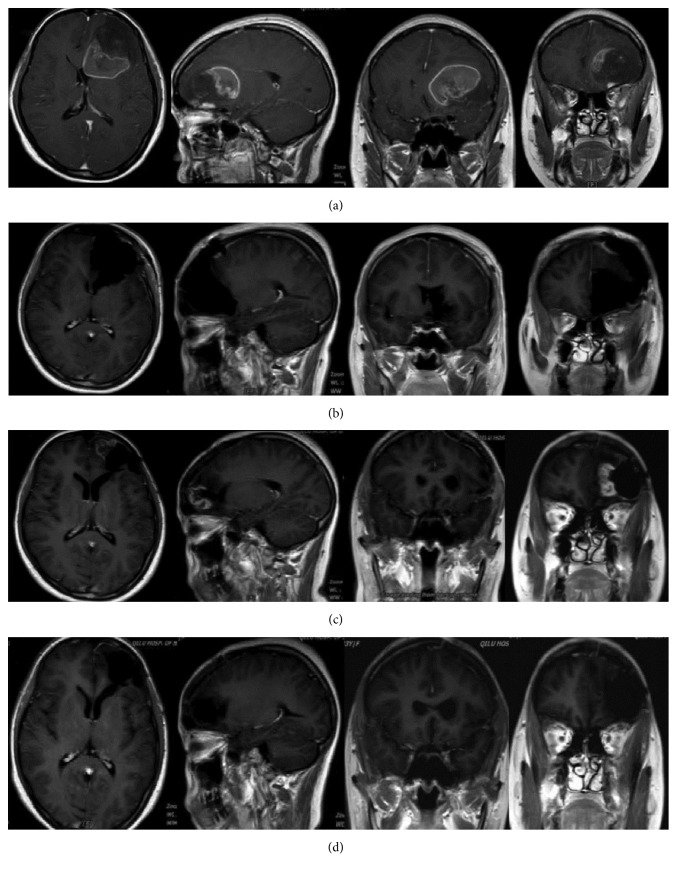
Case (6). (a) Preoperative axial, sagittal, and coronal T1-weighted gadolinium-enhancement MR image revealed a left frontal GBM (tumor volume 51.3 cm^3^). (b) Postoperative (in 72 hours after surgery) axial, sagittal, and coronal T1-weighted gadolinium-enhancement MR image revealed a gross total resection of tumor without any area of CE region. (c) Follow-up axial, sagittal, and coronal T1-weighted gadolinium-enhancement MR image that was obtained 24 months after surgery revealing tumor recurrence. (d) Postoperative (in 72 hours after surgery) axial, sagittal, and coronal T1-weighted gadolinium-enhancement MR image revealed a gross total resection of tumor without any area of CE region.
